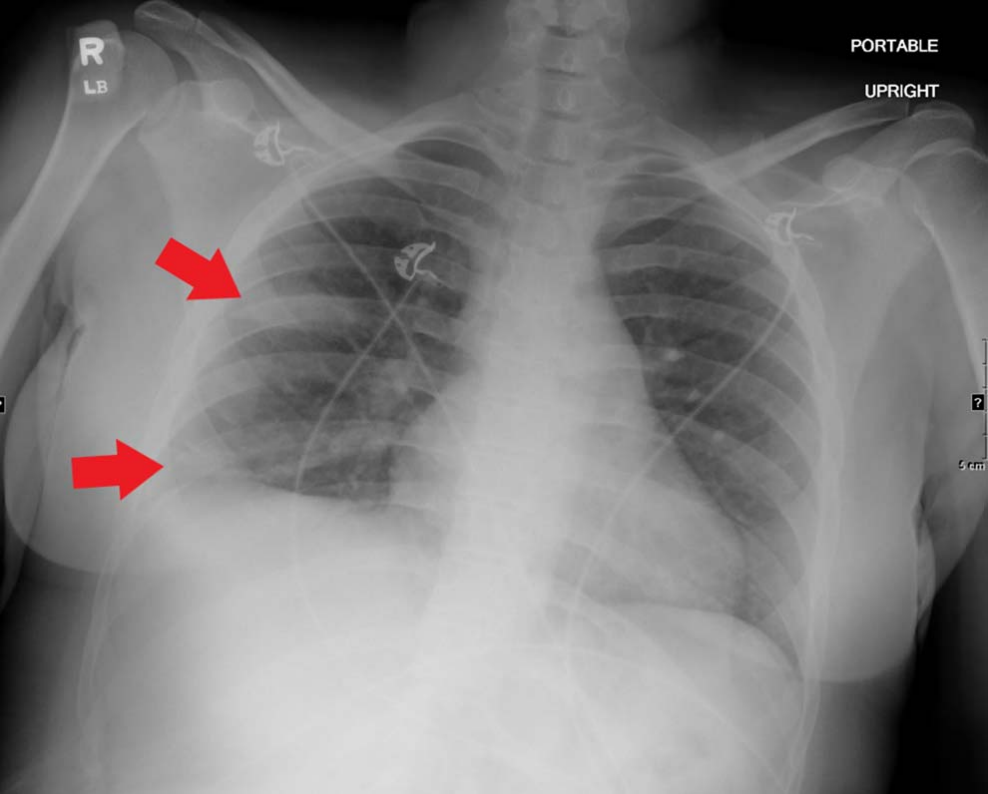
Portable upright anteroposterior radiograph of a 30-year-old female shows unilateral confluent, bandlike (arrows) consolidative opacity in the periphery of the right mid and lower lung zones. This pattern was considered atypical, but more suspicious for coronavirus disease 2019.
